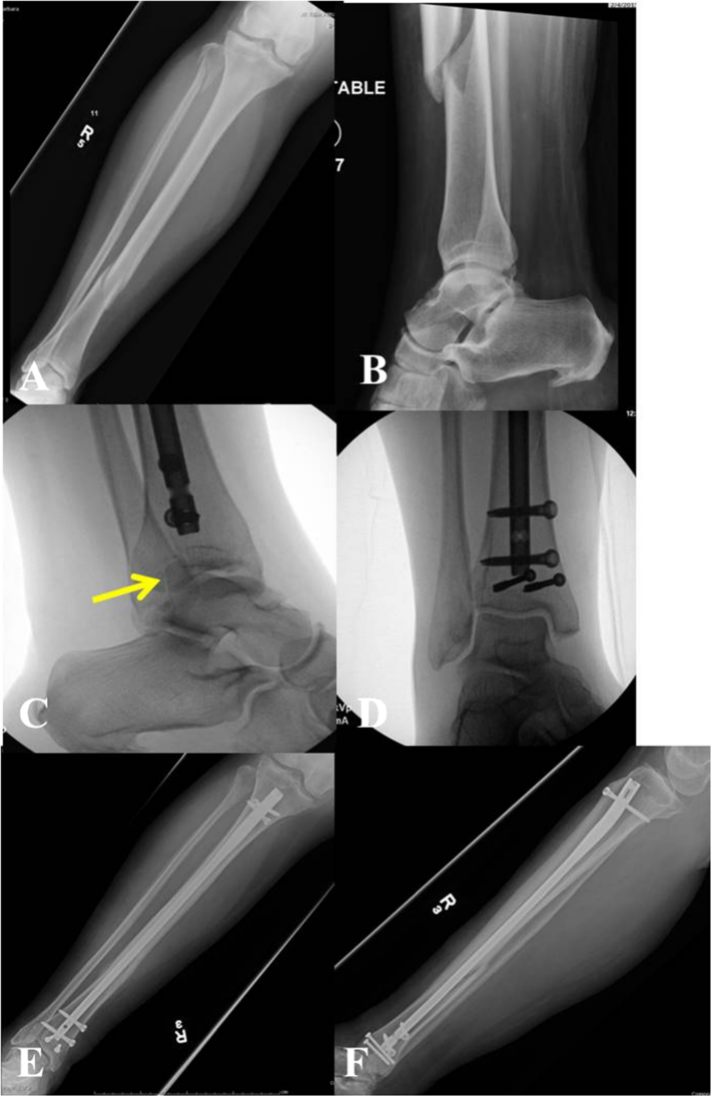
a-f Right distal third spiral tibia fracture (a) with normal appearing preoperative radiographs of the ankle (b). Intraoperative fluoroscopic pictures demonstrating a non-contiguous minimally displaced posterior malleolus fracture (c) requiring surgical fixation (d). Follow-up radiographs (e-f) show uneventful healing of both tibia and ankle injury
